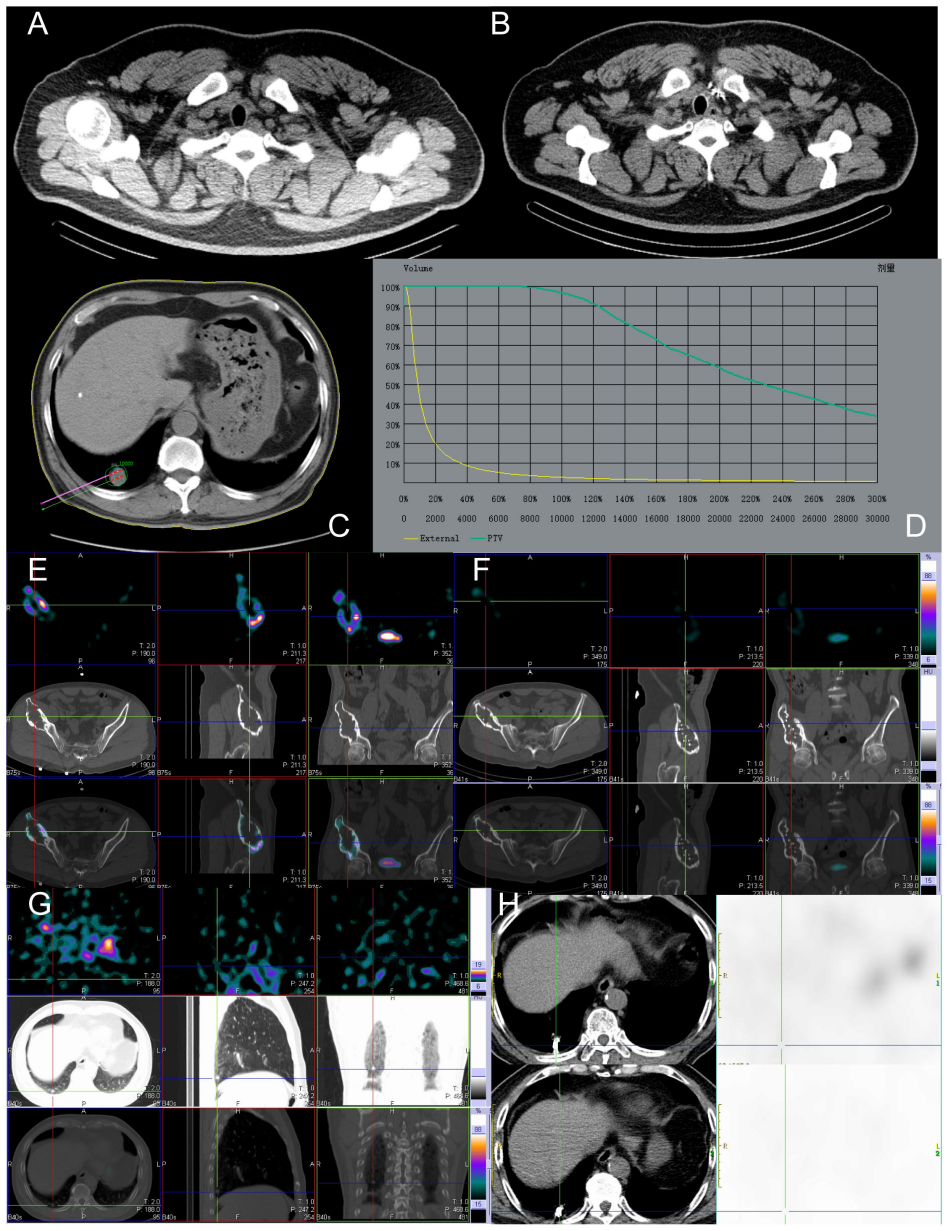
Before and after implantation of left supraclavicular lymph node, lung, and right ilium lesions with ^125^ I seeds. The patient, a 49-year-old male with RAIR-DTC, had postoperative pathologic findings of papillary thyroid carcinoma. (A) Images of ^125^ I seeds before implantation. (B) CT reexamination 12 months after the implantation of ^125^ I seeds showed only the shadow of metal seeds. (C) Preoperative needle placement of TPS system. (D) After the dose verification, the planned target DVH showed D_90_ > 100 Gy (prescription dose) and V_200_ < 60%, indicating satisfactory dose distribution of particle implantation with no “cold area” or “hot area” in dosimetry. (E) Single Photon Emission Computed Tomography/Computed Tomography (SPECT/CT) revealed iodine uptake in the right ilium metastatic lesion. (F) After 12 months of treatment, compared to before treatment, the radiation uptake significantly decreased, indicating effective local treatment, partial inhibition of tumor activity, and significant improvement in the patient’s bone pain. (G) No iodine uptake was found in the right lower lobe lung metastasis by SPECT/CT. (H) After 12 months of treatment, CT images showed a significant reduction in the mass and partial relief in the local effect. Tg, Thyroglobulin; RAIR-DTC:Radioiodine-refractory differentiated thyroid cancer; CT, Computed Tomography; TPS, Treatment planning system; DVH, Dose-volume histogram; SPECT/CT, Single Photon Emission Computed Tomography/Computed Tomography.
